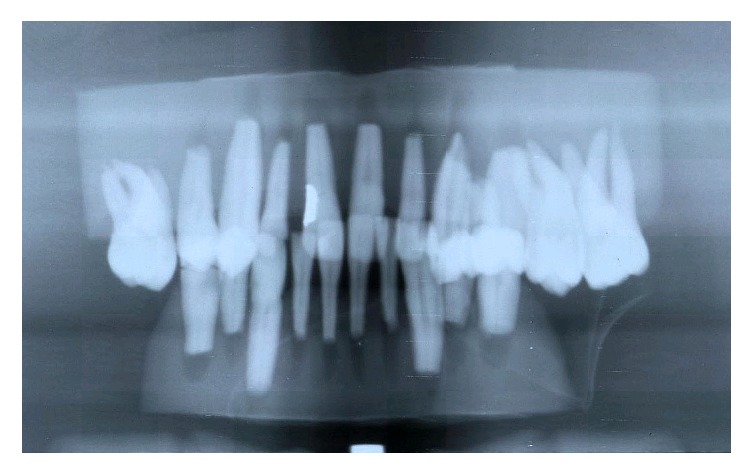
Panoramic radiograph: rizalysis.
